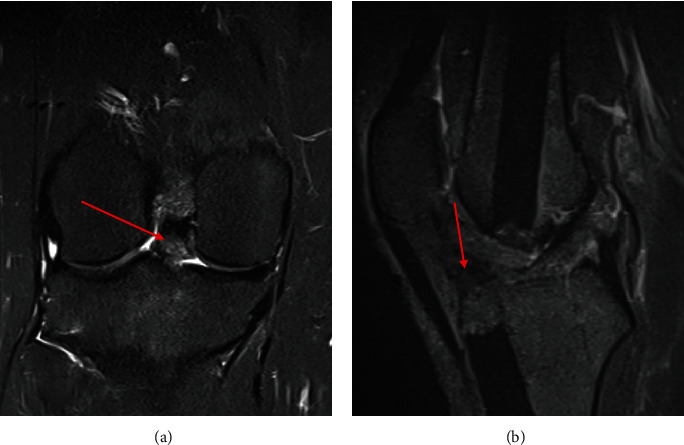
Preoperative right knee magnetic resonance imaging study. The red arrows indicate the anterior intra-articular heterotopic ossification: (a) select coronal T2 image; (b) select sagittal T2 image. A red arrow indicates the anterior intra-articular heterotopic ossification in both images.
